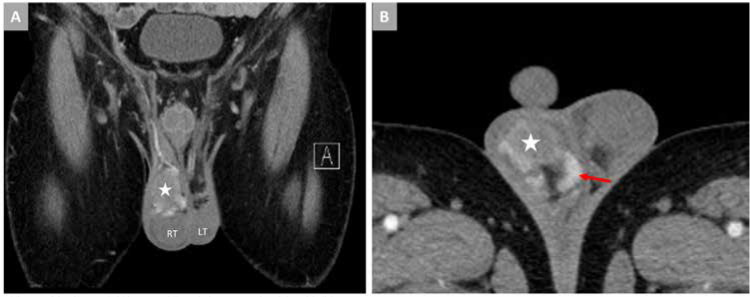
(A) Coronal CT scan of the abdomen and pelvis with IV contrast: Mass (3.7 x3x2.4 cm) in the distal right spermatic cord seen above the right testis. Asterisk: mass; RT: right testis; LT: left testis. (B) Axial CT section at the level of mass lesion. Red arrow: contrast enhancing varicoceles.
